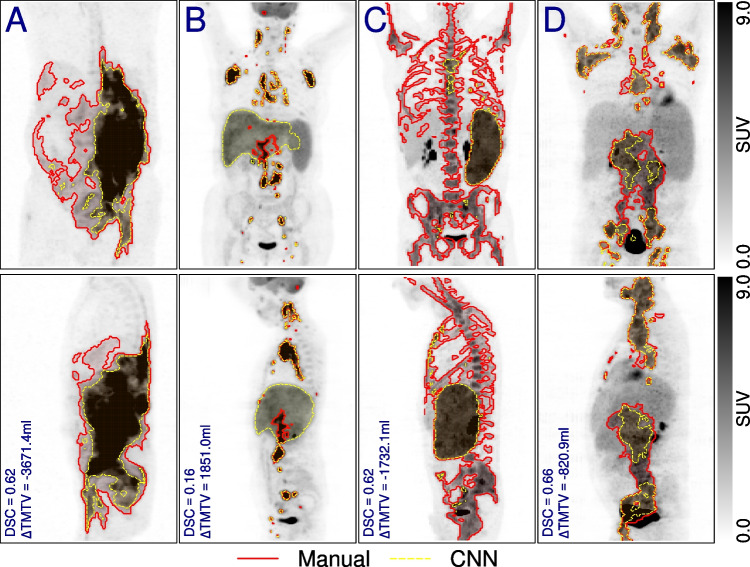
Examples of the outliers in Fig. 2 from the cross-validation experiment. These studies represent the most frequent reasons for large deviations in TMTV between automated and manual delineations. Patient A: diffuse intestinal and mesenterial uptake; patient B: false inclusion of liver with elevated [^18^F]FDG uptake; patient C: false negative bone uptake; patient D: partial omission of diffuse uptake in confluent retroperitoneal lymphoma. Coronal (top) and sagittal (bottom) maximum intensity projections of PET images are shown
